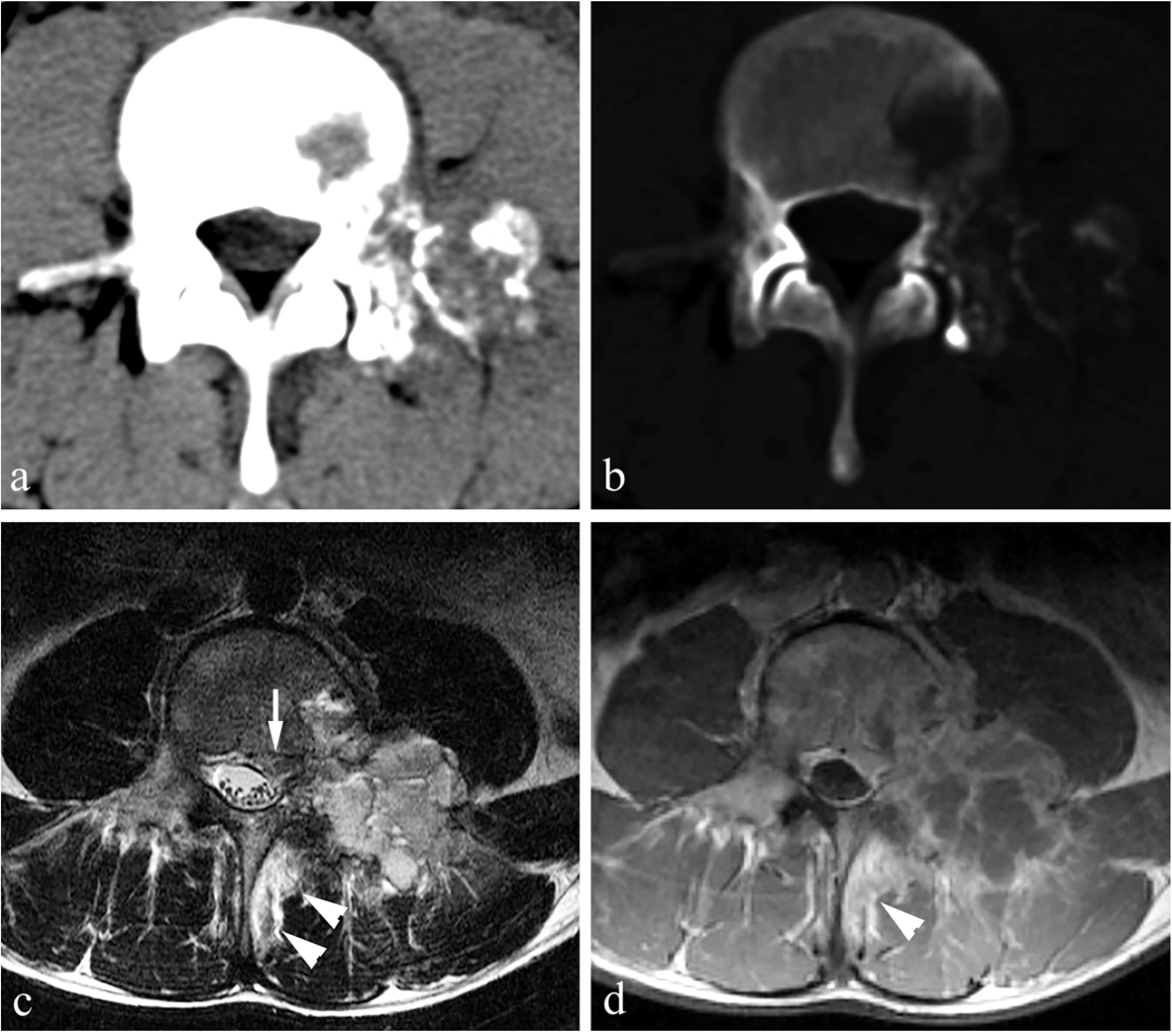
Osteoblastoma in a 29-year-old male, Enneking stage 2 on CT and stage 3 on MRI. a Axial CT bone window shows expansile bone destruction in the left lamina of L7 with a clear sclerotic rim and speckled calcifications. Note sclerotic changes (arrows) in the surrounding areas. Axial MRI T2WI (b), T1WI (c), and contrast-enhanced T1WI (d) show a soft tissue mass in the epidural space (arrowheads)
